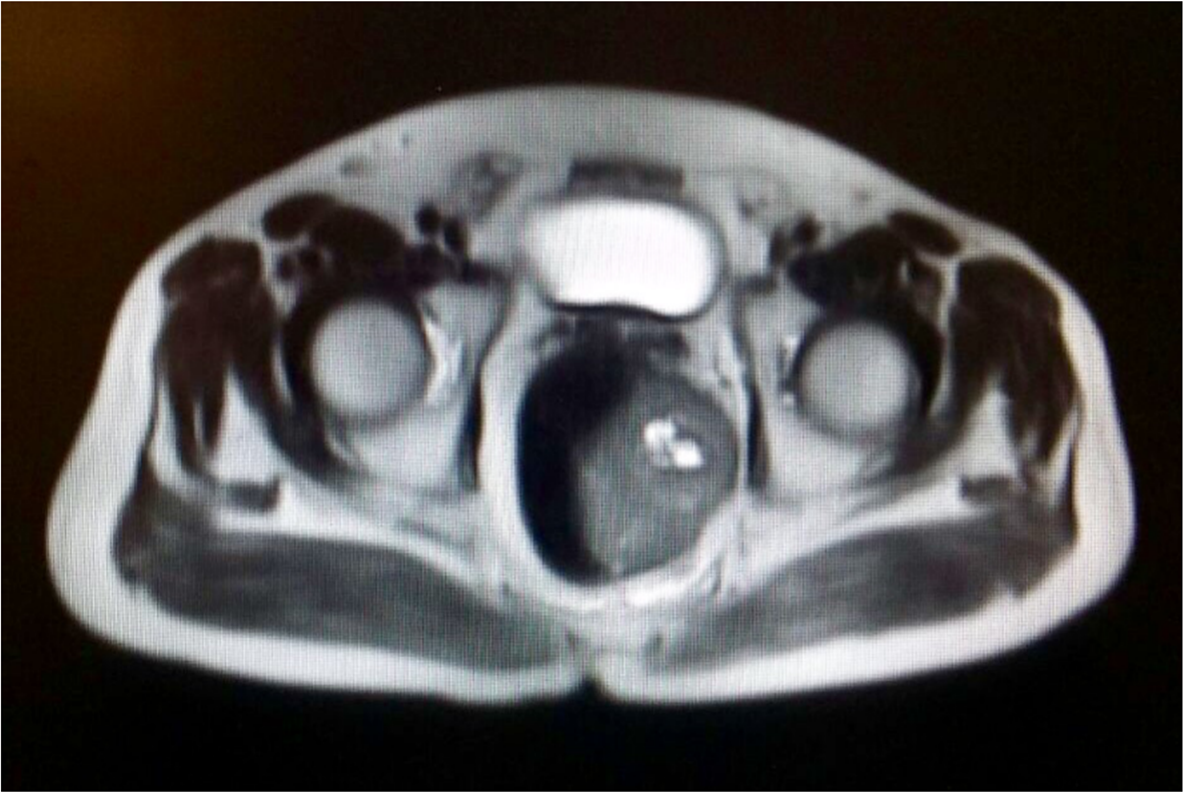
Axial T1-weighted magnetic resonance image shows a well-defined round mass, with low signal intensity on T1-weighted image and on T2-weighted image, arising from the distal rectum
